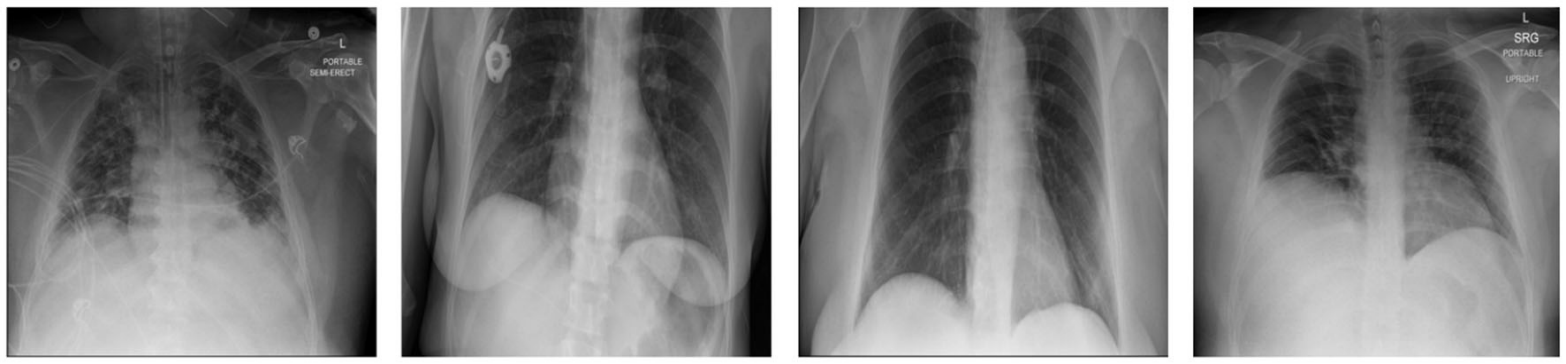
Example chest X-ray images from the benchmark dataset.
